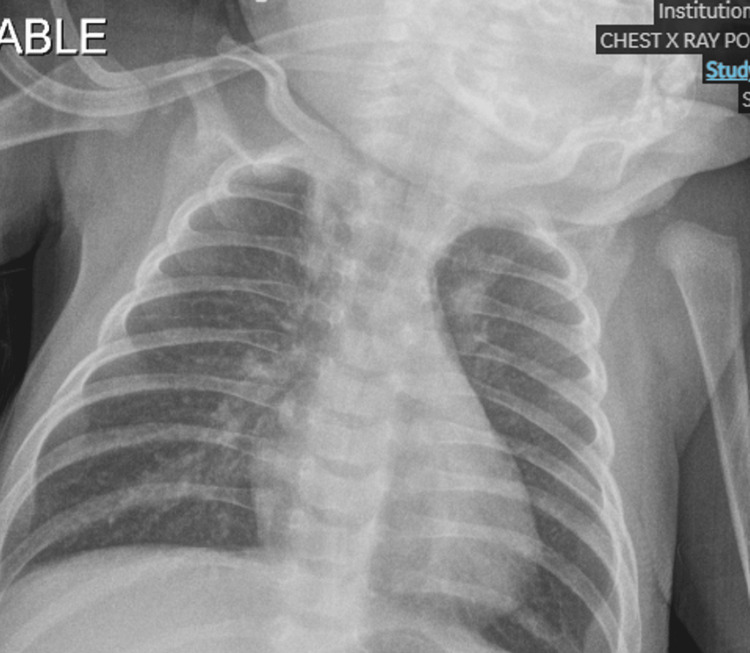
Chest radiograph after the second extubation showing well‑expanded lungs without pleural effusion or new consolidation.
